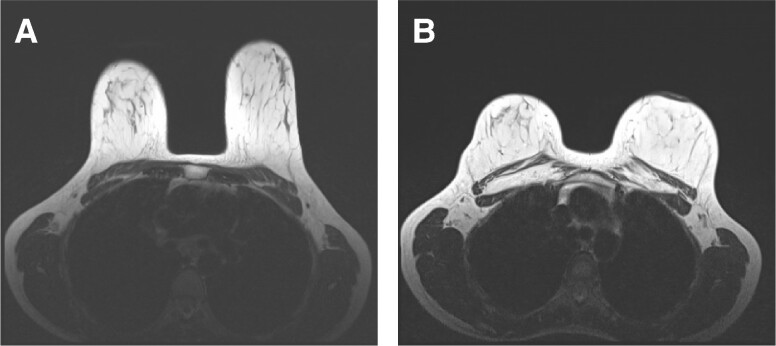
Exemplary MRI slices of the aforementioned patient. A 34-year-old female patient underwent a reduction/augmentation-mastopexy procedure, and received autologous fat transfer to the breast. (A) Before the surgery. (B) 19 months after the surgery.
